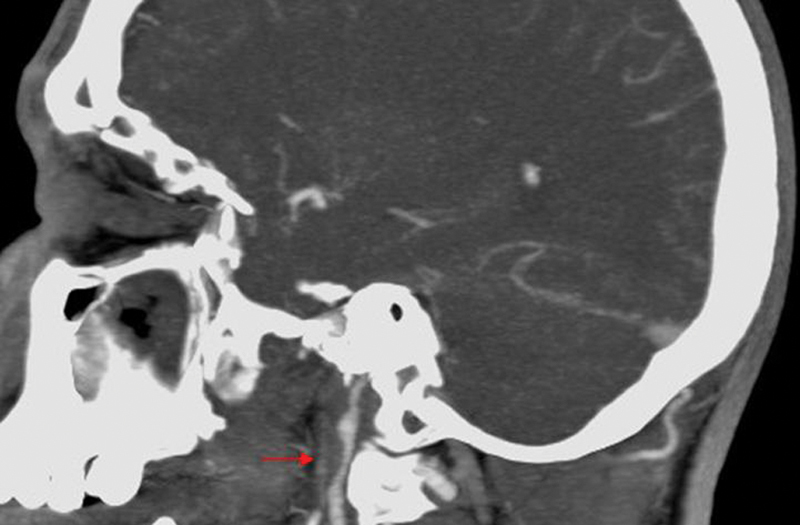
Computed tomography angiography demonstrates the presence of carotid dissection and pseudoaneurysm (arrow).
